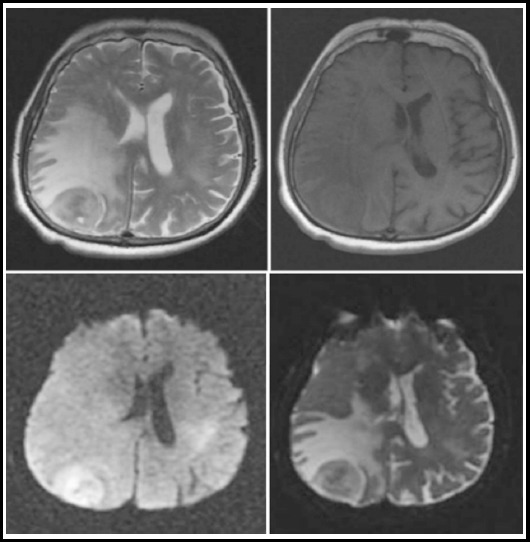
Axial T2WI, T1WI, DWI and ADC images reveal a malignant posterior parietal meningioma showing diffusion restriction on DWI/ADC and marked peritumoral edema.
